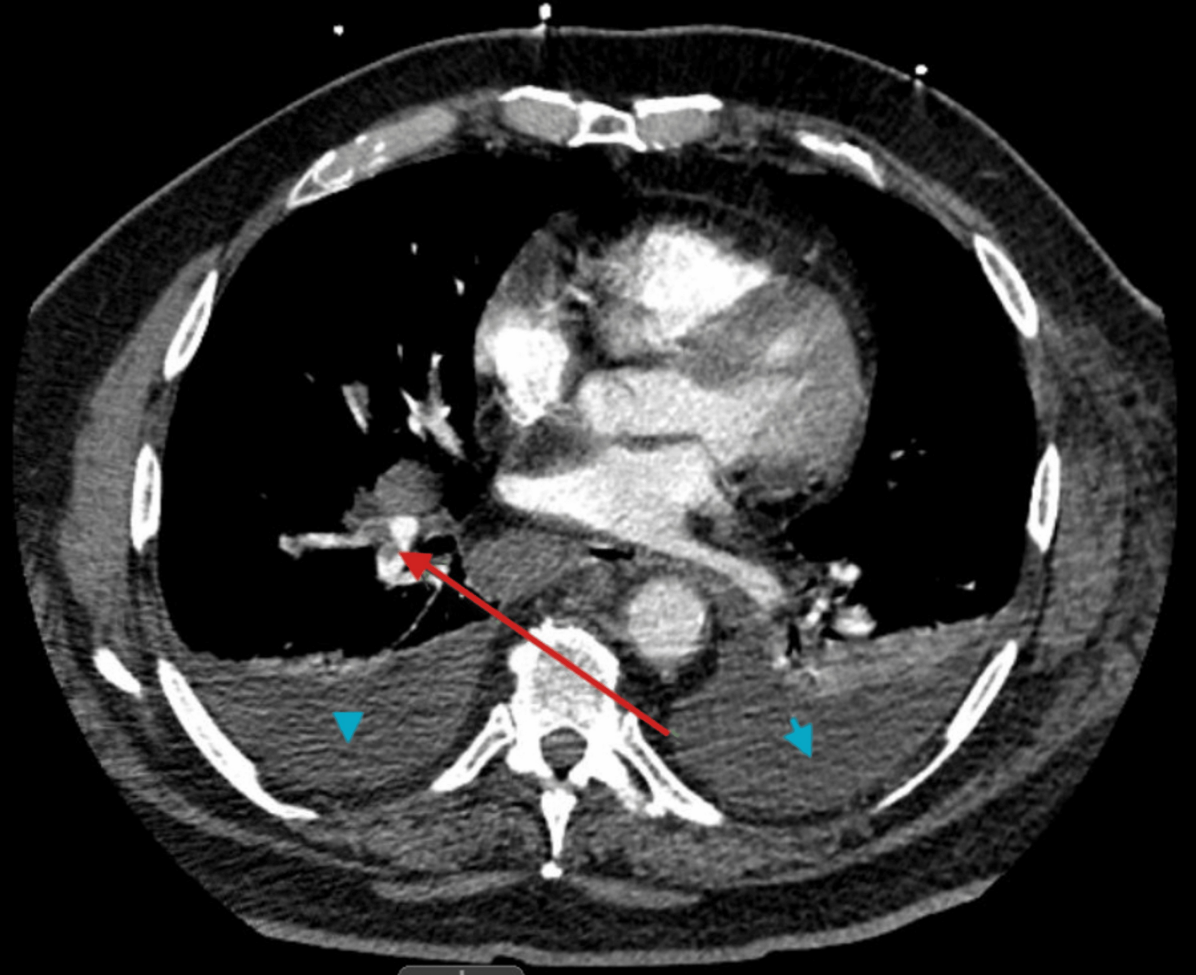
Computed tomography pulmonary angiogram axial image showing filling defect consistent with pulmonary embolism marked by red arrow and blue arrow heads showing bilateral pleural effusion.
